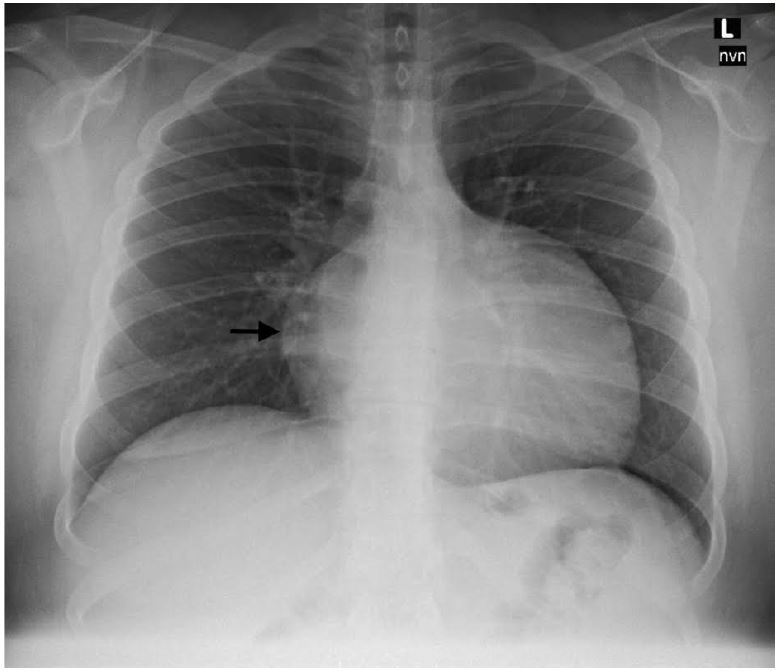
Chest radiograph showing enlarged cardiomediastinal silhouette with narrow vascular pedicle.
